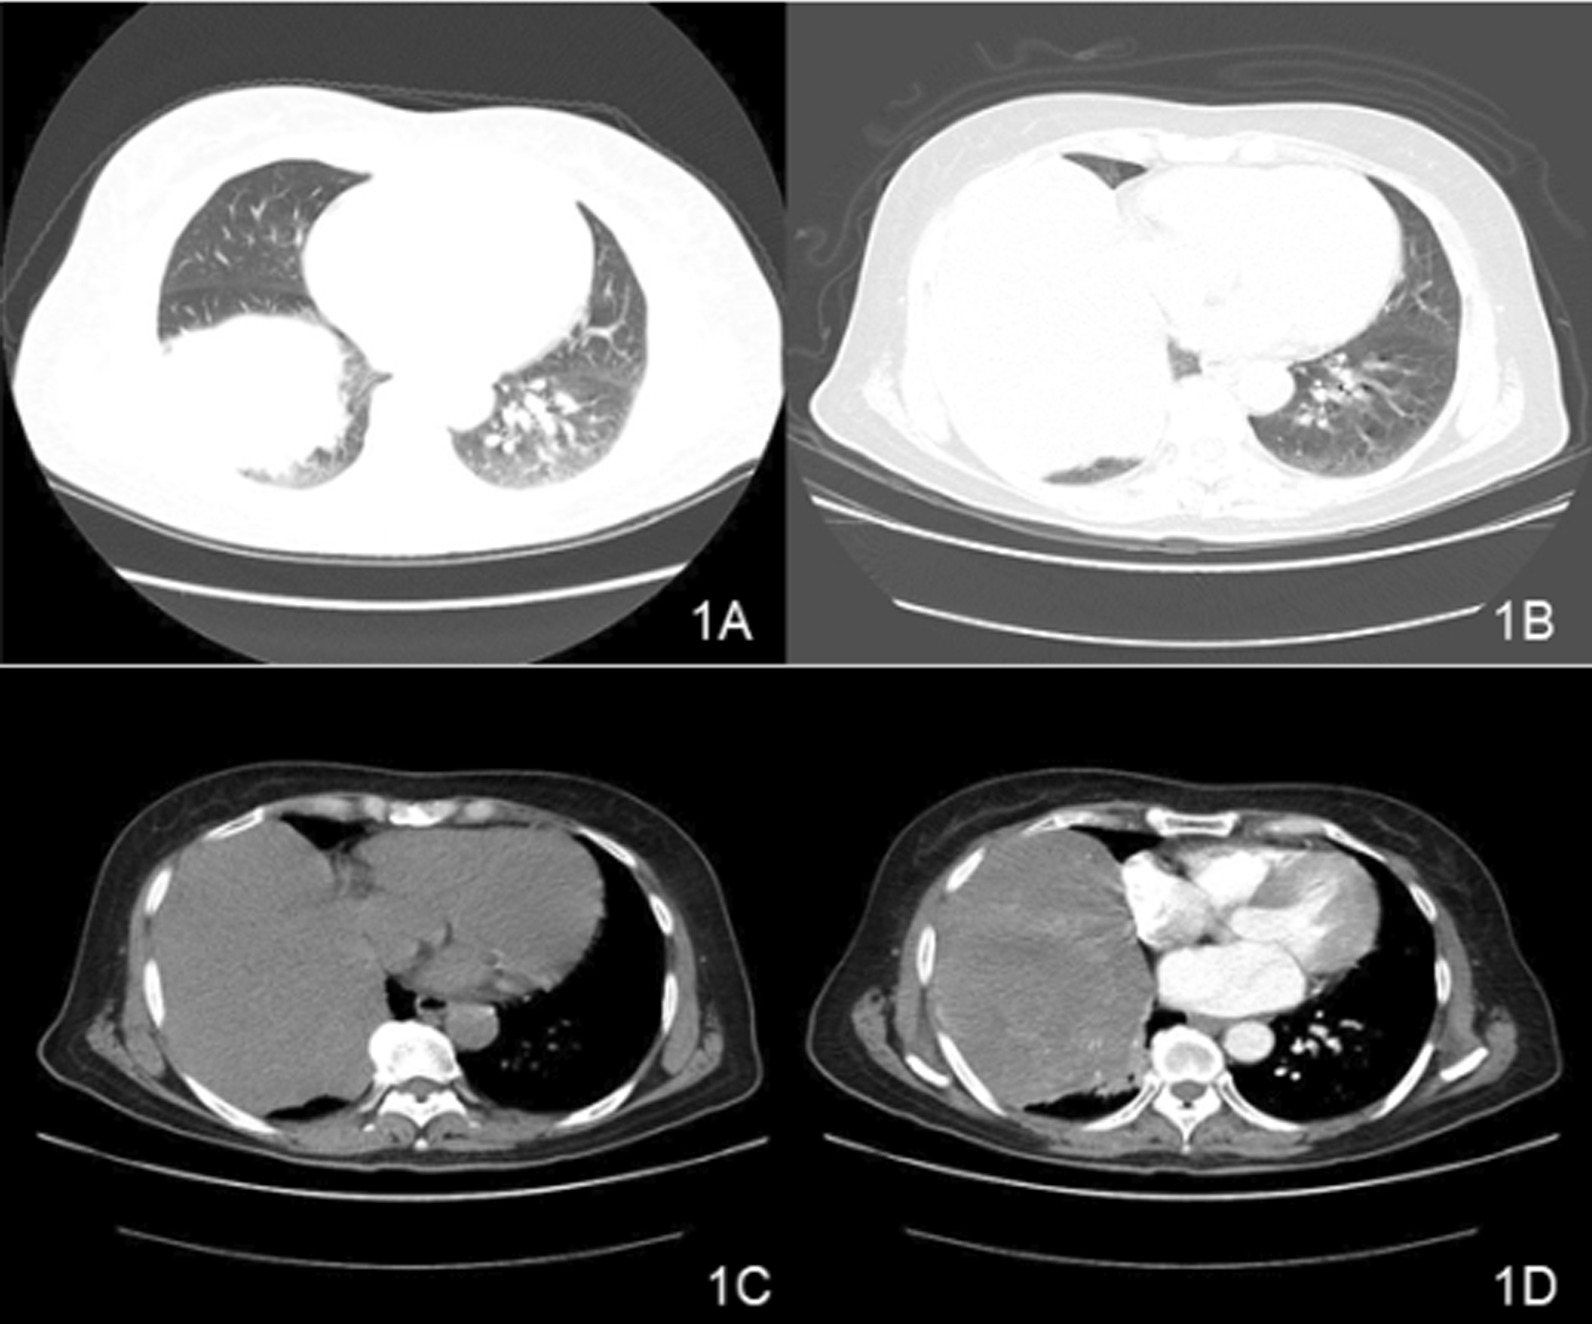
A The CT film from 2007. B The CT film from 2017 showing the axial lung window; the mass was significantly larger than before. C and D Large soft tissue mass at the bottom of the right chest, approximately20cm × 13 cm × 12 cm in size, with a clear border and significant enhancement at the axial mediastinal window
